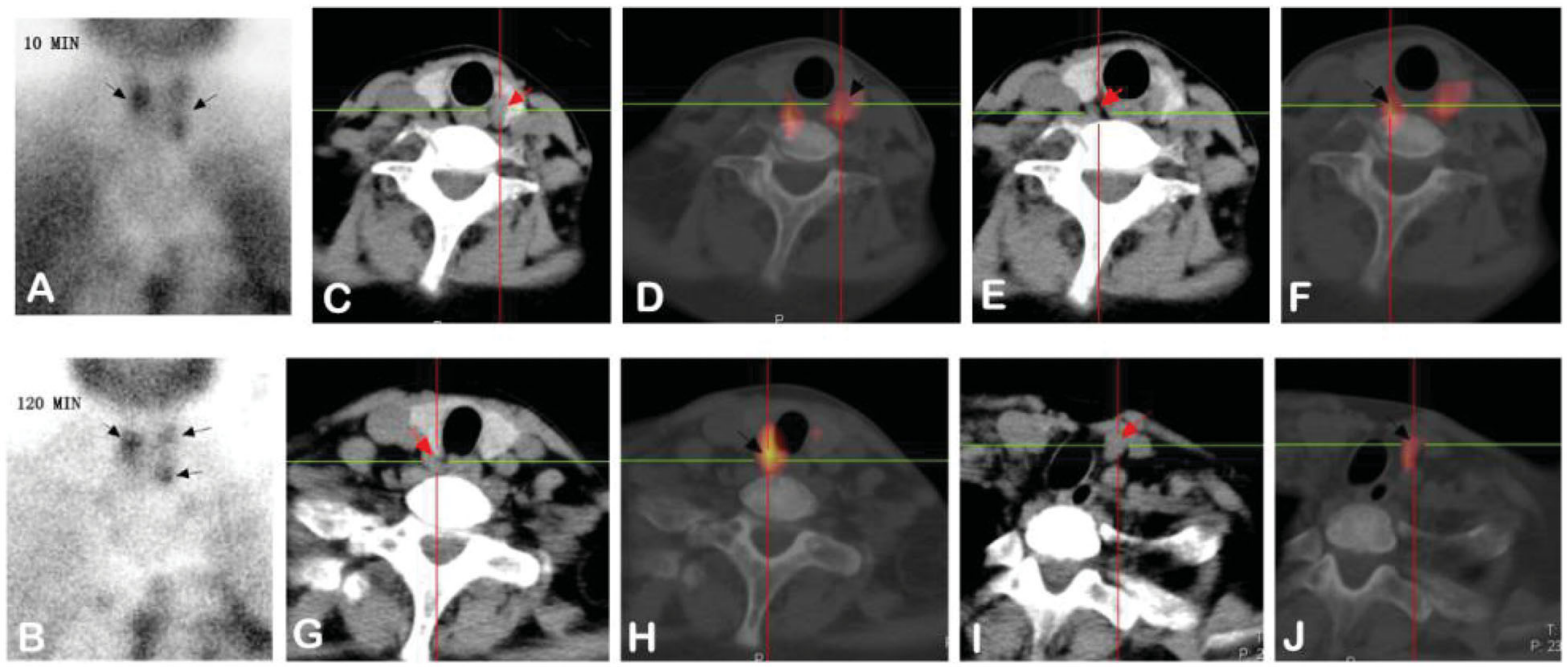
Images from a 28-year-old female diagnosed with SHPT. (A,B): Dual phase 99mTc-MIBI planar scintigraphy of parathyroid glands. (A) early phase (after 10 minutes post injection of tracer) – showing radioactivity uptake in thyroid with increased distribution in left lobe and upper pole of right lobe. (B) late phase (after 120 min) – activity of wash-out from thyroid and retention in hyperfunctioning parathyroid glands (upper pole and inferior pole of left thyroid, upper pole of right thyroid, black arrows). (C–H): CT alone (C/E/G) and 99mTc-MIBI SPECT/CT images (D/F/H) of the same cross-section views showing the right upper (C and D), the left upper (E and F) and inferior (G and H) hyperfunctioning parathyroid glands (arrows). (I,J): CT alone (I) and 99mTc-MIBI SPECT/CT images (J) of the same cross-section views showing a new hyperfunctioning parathyroid gland that was not found in 99mTc-MIBI planar imaging in the inferior pole of right thyroid (arrows).
